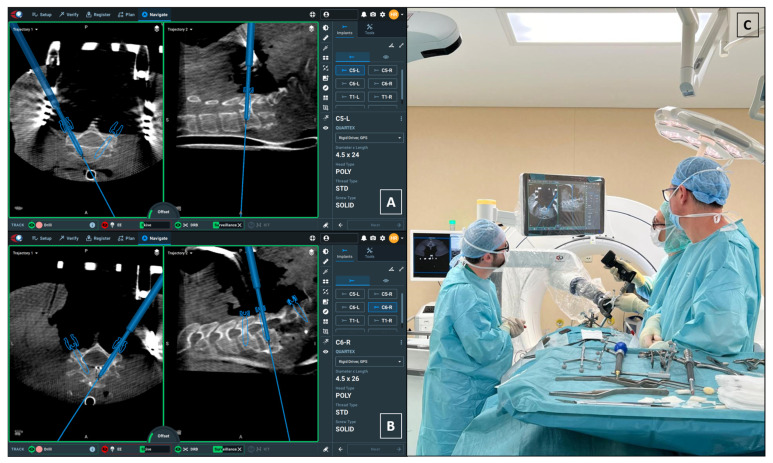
Intraoperative robotic planning and surgical setup. (A,B) Interface screenshots of the ExcelsiusGPS® navigation system during trajectory planning for the left C5 (A) and right C6 (B) pedicle screws. Each panel displays the corresponding axial (left sub-panel) and sagittal (right sub-panel) projections. The axial views clearly demonstrate the strategic planning: while the left side allows for a standard trajectory, the right-side trajectories (visible in panel (B) and as the contralateral plan in panel (A)) show the intentional, small, controlled violation of the medial pedicle cortex (medial breach). This strategy was employed to accommodate the 4.5 mm screw diameter while strictly avoiding the lateral vertebral artery canal. (C) Intraoperative photograph showing the surgical field, the robotic arm, and the O-Arm™ imaging system. Crucially, the registration CT scan was acquired with the cervical self-retaining retractors already secured in place; this technical nuance prevents vertebral realignment or shift occurring after imaging, ensuring that the navigation data perfectly matches the real-time anatomy during screw placement. In these navigation views, orientation is ipsilateral (right displayed on the right, left on the left).
